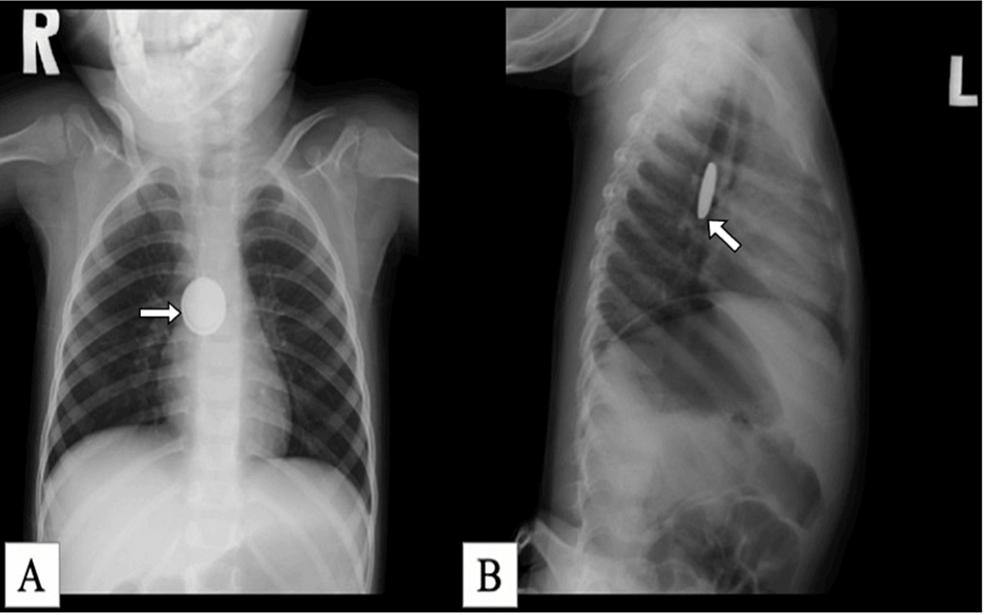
The chest and abdominal X-ray (A) The anteropesterior (AP) view revealed double ring like disc shaped radiopaque shadow in the middle third of esophagus; (B) the lateral view showed increase thickness and groove (arrow) foreign body, posterior to airway tract.
